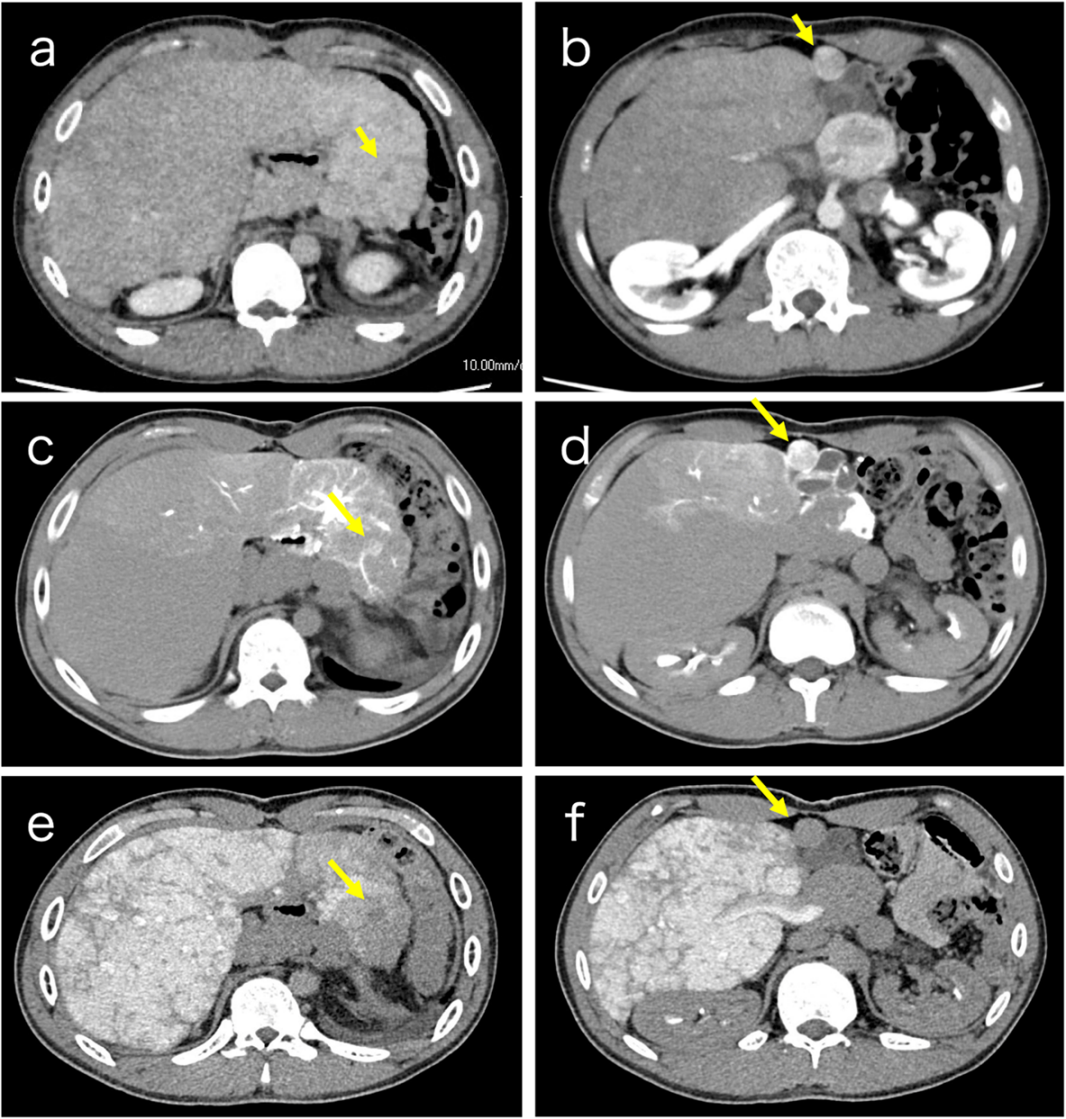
Abdominal contrast-enhanced CT and CT arteriography and arterial portography. Abdominal contrast-enhanced CT examination revealed a hypovascular mass in segment 2 (a), and a hypervascular mass in segment 4 on arterial phase, followed by delayed washout (b). CT arteriography showed that both masses were hypervascular tumors (c: segment 2, d: segment 4), and CT during arterial portography showed that both were low-density masses (e: segment 2, f: segment 4)
